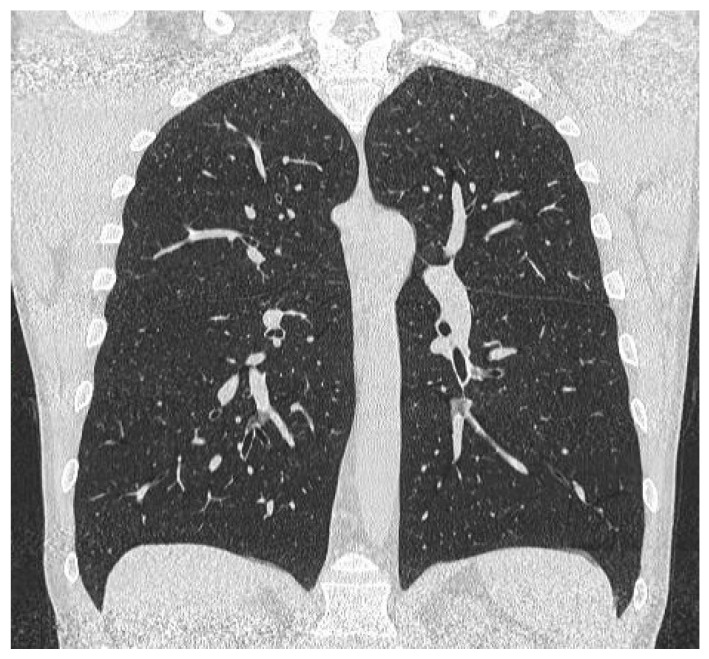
Coronal reconstruction of the unenhanced chest CT scan performed in 2021 during the SARS-CoV-2 infection. Diaphragms are symmetrical without any signs of elevation.
